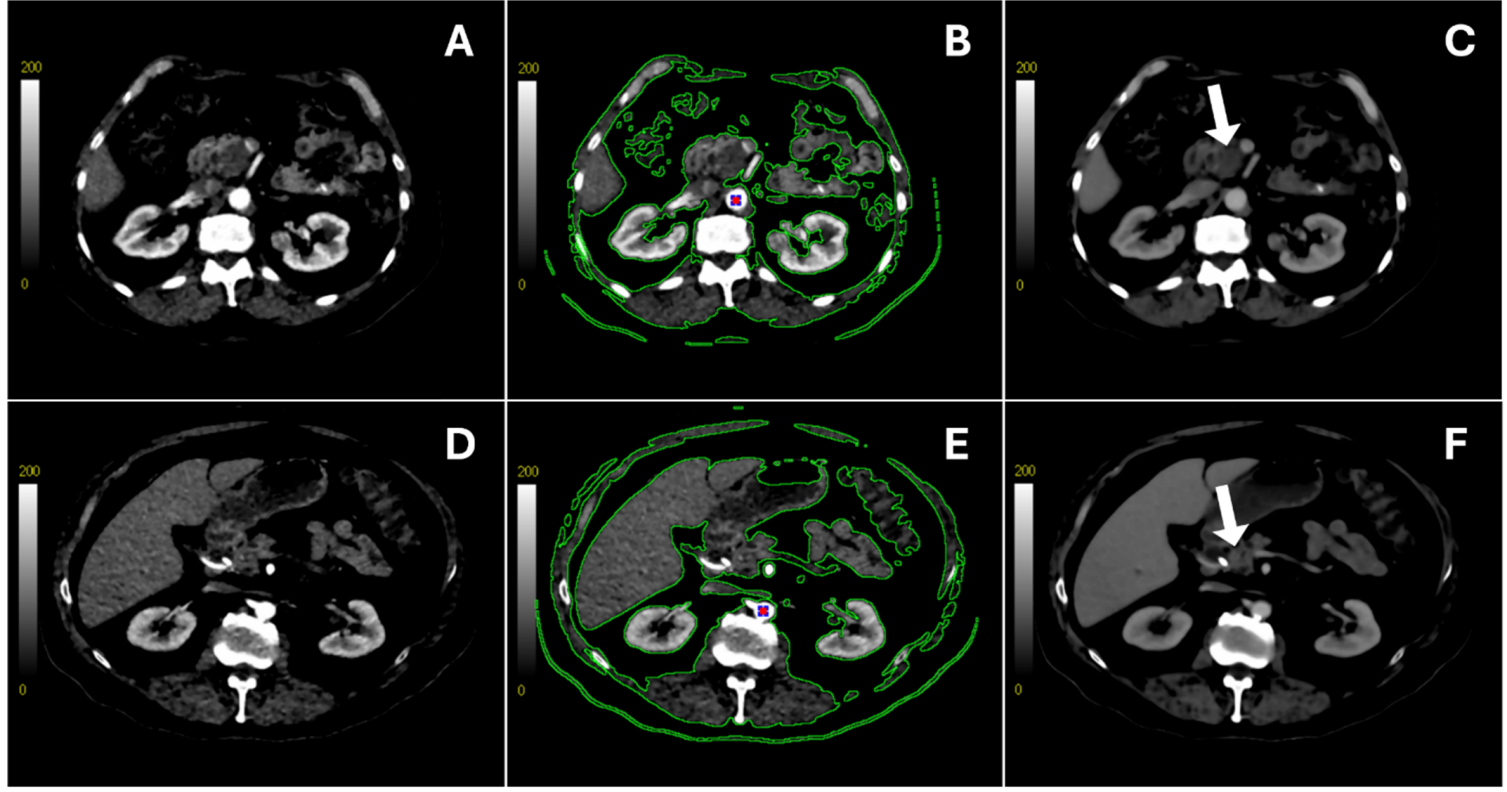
The top and bottom rows show the contrast-enhanced CT scan of one of the axial slices obtained from a borderline resectable patient (BRPC, Patient 2) and the resectable patient (RPC), respectively. Scans in the left column were acquired shortly after contrast injection (A, D). The same scans are also shown in the middle column, with the segmented region enclosed in green showing where the deconvolution algorithm was applied to generate the perfusion maps (B, E). The red dot shows the 3 x 3 pixel region of interest from which the arterial time-density curve (TDC) was obtained. The right column shows the average CT scan of the same axial slice (C, F). The white arrow is pointing at the gross tumor volume (GTV).
